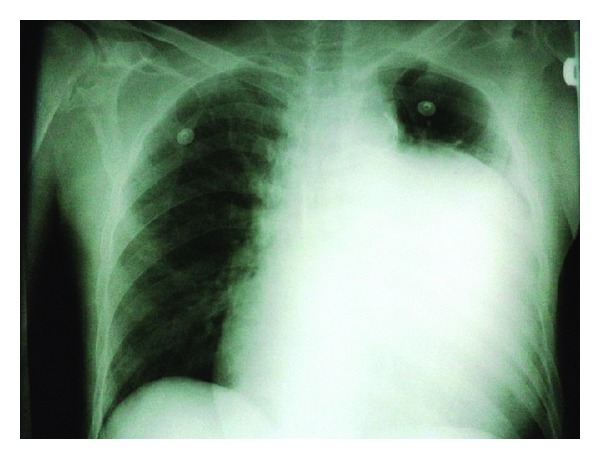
Patient's chest radiography shows cardiothoracic enlargement with homogenous opacity in left hemithorax. Space occupying, cystic lesions, and vascular aneurysm are differential diagnosis of this condition.
